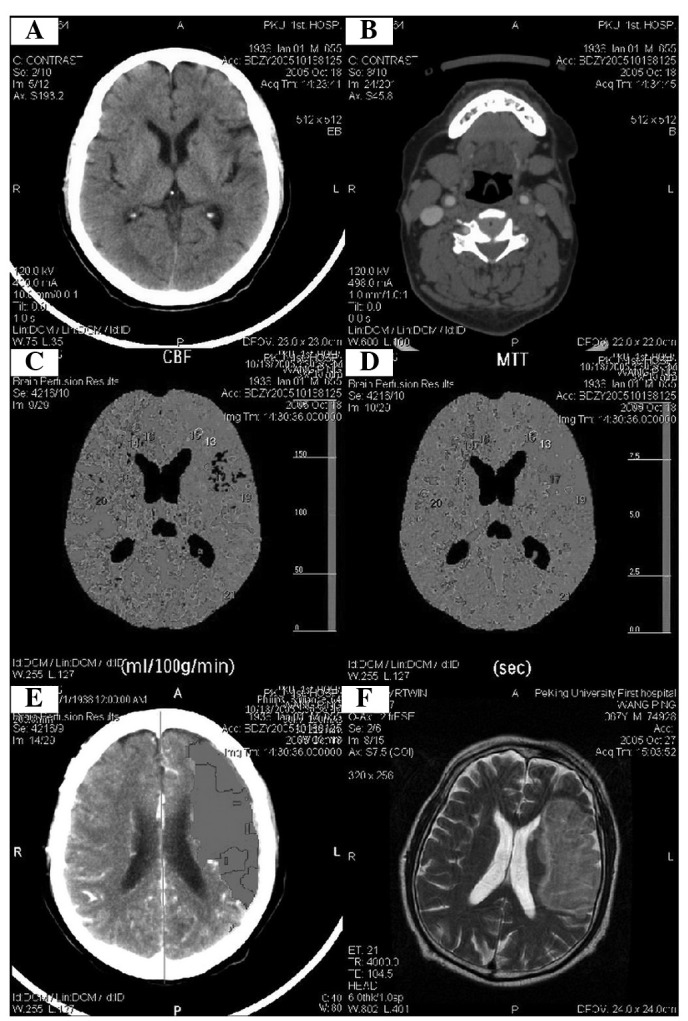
Case 2, male, 67 years old. Language was not fluent and the mouth/nose was skewed when admitted into hospital. The patient presented mixed aphasia with a consciousness trance-like state for 3 h and the right pathology was weak positive. The plain computed tomography (CT) scan revealed that a lacunar infarct occurred in the left frontal hemisphere, head of the left caudate nucleus and the left radiation crown area. However, CT perfusion identified large areas of ischemia in the left hemisphere and a small infarction area in the middle hemisphere. Two days later, limb function gradually accelerated, until complete paralysis and aphasia occurred. (A) New cerebral infarction in the head of the caudate nucleus. (B) Bilateral carotid atherosclerotic stenosis on the left (50%) and right (30%). (C and D) The cerebral blood flow (CBF) ratio around the lesion core of the left hemisphere was <0.2 indicating irreversible ischemic infarction and the area surrounding the core was considered the ischemic penumbra. (E and F) The infarct area was observed after <3 days using magnetic resonance imaging (MRI).
